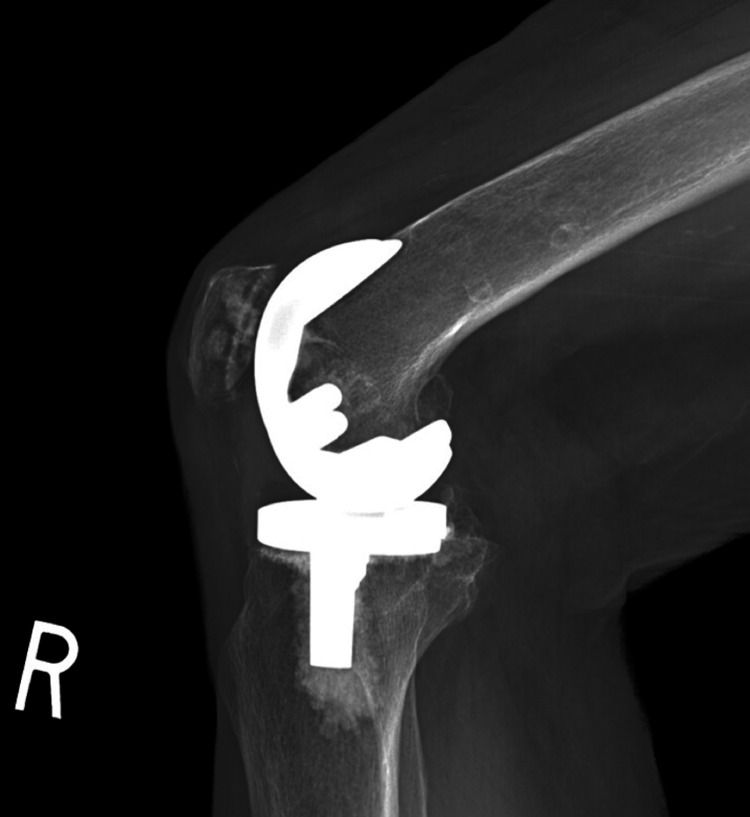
Lateral radiograph of the knee demonstrating absence of the linear lucency five years after surgery
